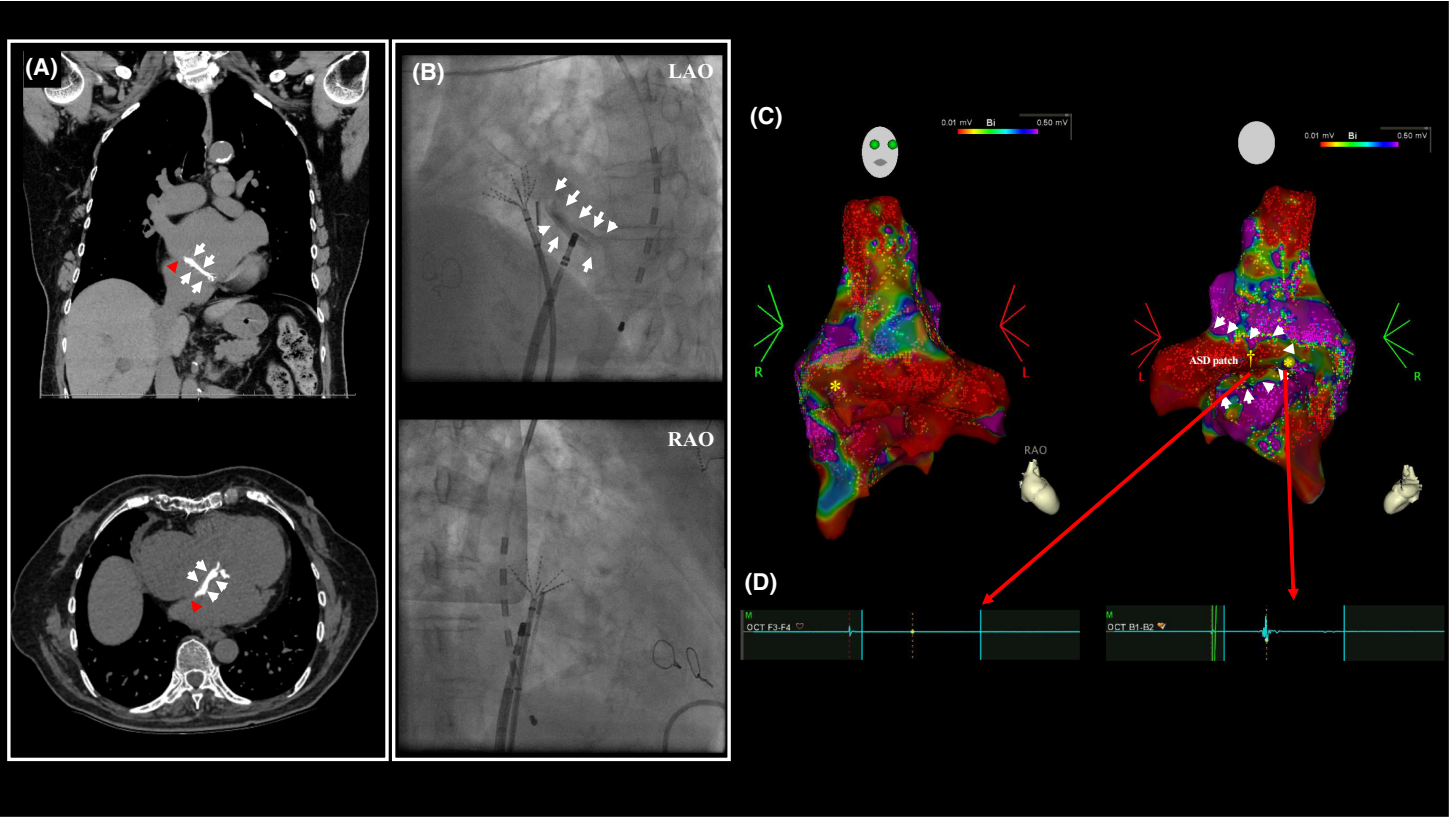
(A) A computed tomography revealed a highly calcified septum (white arrow) in which the residual native septum length was less than 1 cm (red arrowhead). (B) Fluoroscopic image showing a highly calcified septum (white arrow). The ablation catheter was placed onto the native atrial septum based on the 3D map and SOUND STAR image. (C, D) The voltage map showed a large scar zone (dagger) due to the septal patch (white arrow), and the surrounding native interatrial septum is shown as a low‐voltage area (asterisk).
